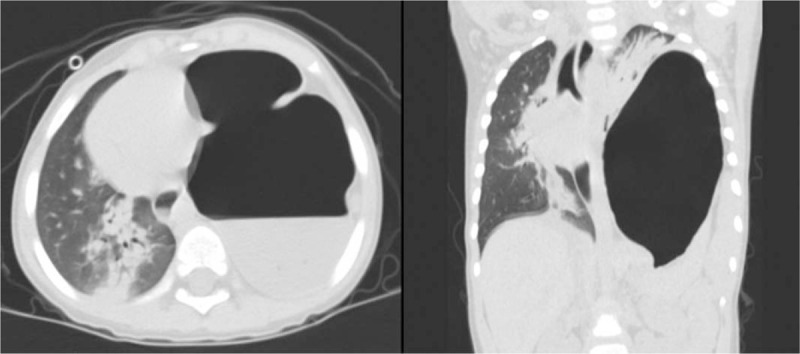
Chest computed tomography (CT) scan showing the stomach herniated through the diaphragmatic defect with significant distension with fluid and air, leading to total collapse of the left lung and shifting of the mediastinum shifting, as well as right-sided pneumonia.
